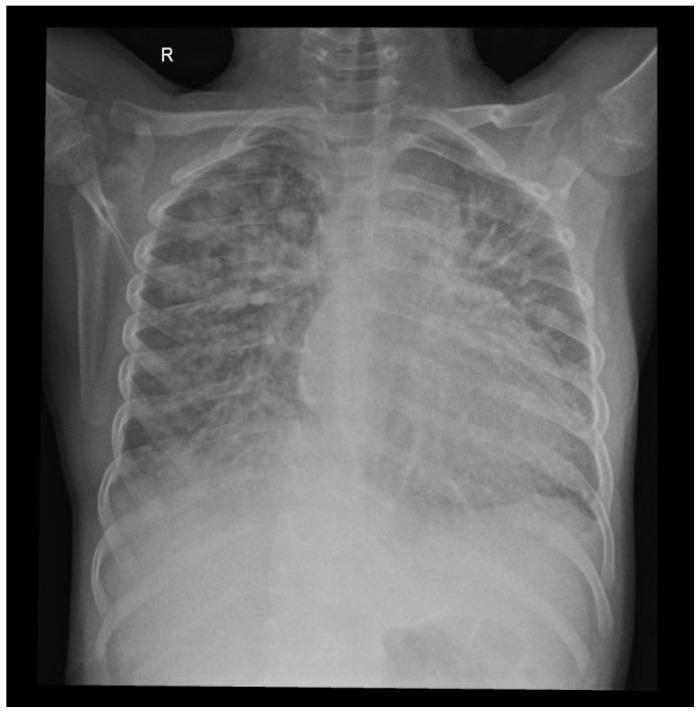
Chest X-ray: pulmonary edema, bilateral diffuse alveolar opacities and cardiomegaly.
